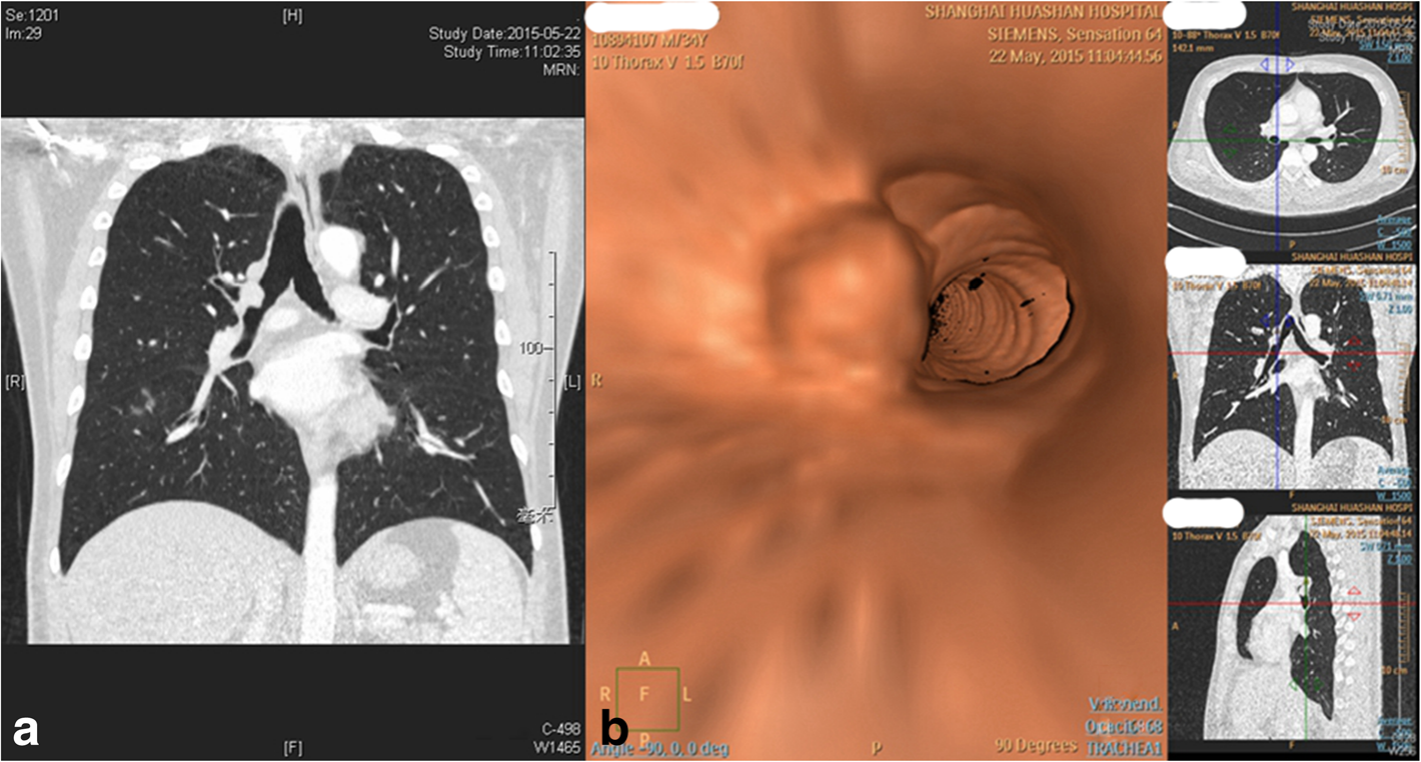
a Multi-planar reconstruction (MPR) by chest CT showing a mass approximately 1.3 cm in diameter at the onset of right upper lobar bronchus; b Virtual bronchoscopy showing a neoplasm protruding from the right upper lobar bronchus to the main bronchus
